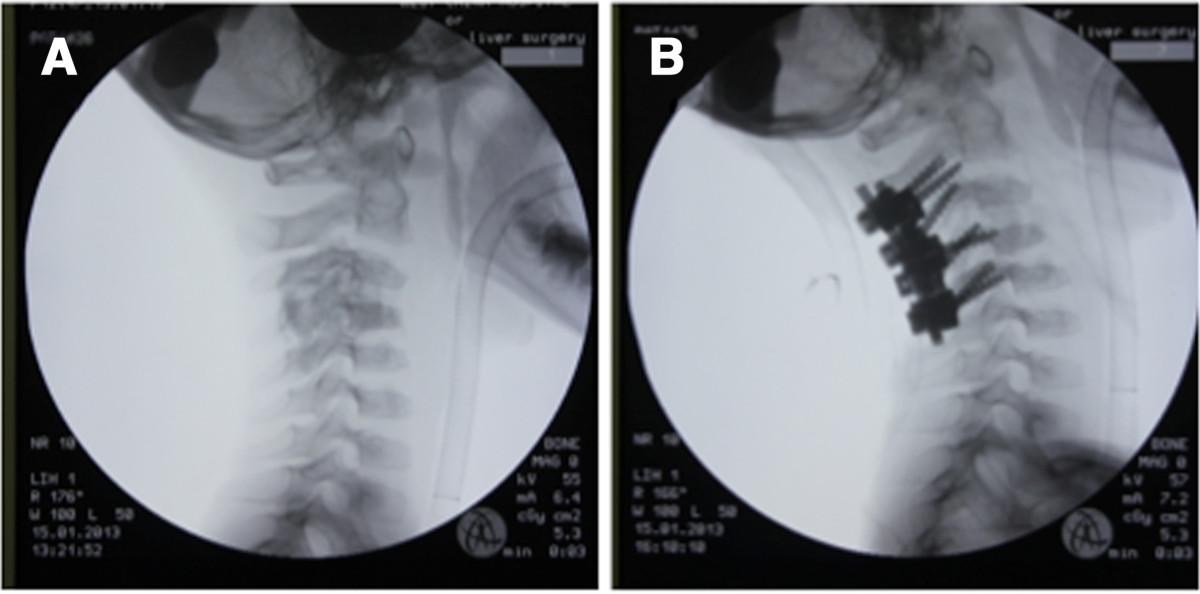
Intraoperative radiograph shows no spinal instability after anesthesia was induced and the Mayfield clamp was positioned (A), and the result of the reconstructive technique (B).
